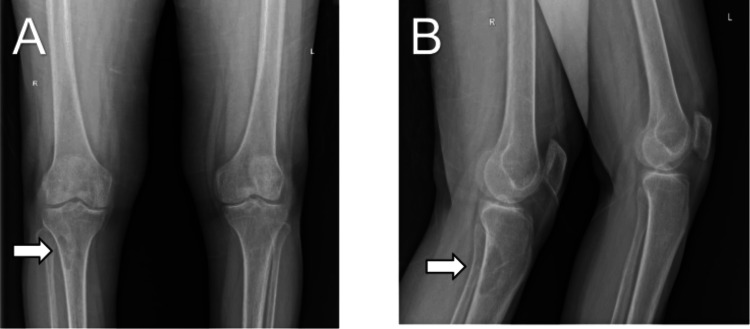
An X-ray of bilateral knee joints showing osteolytic lesion (arrow) in the posterolateral aspect of the right tibia: panel A (anteroposterior view) and panel B (lateral view).
